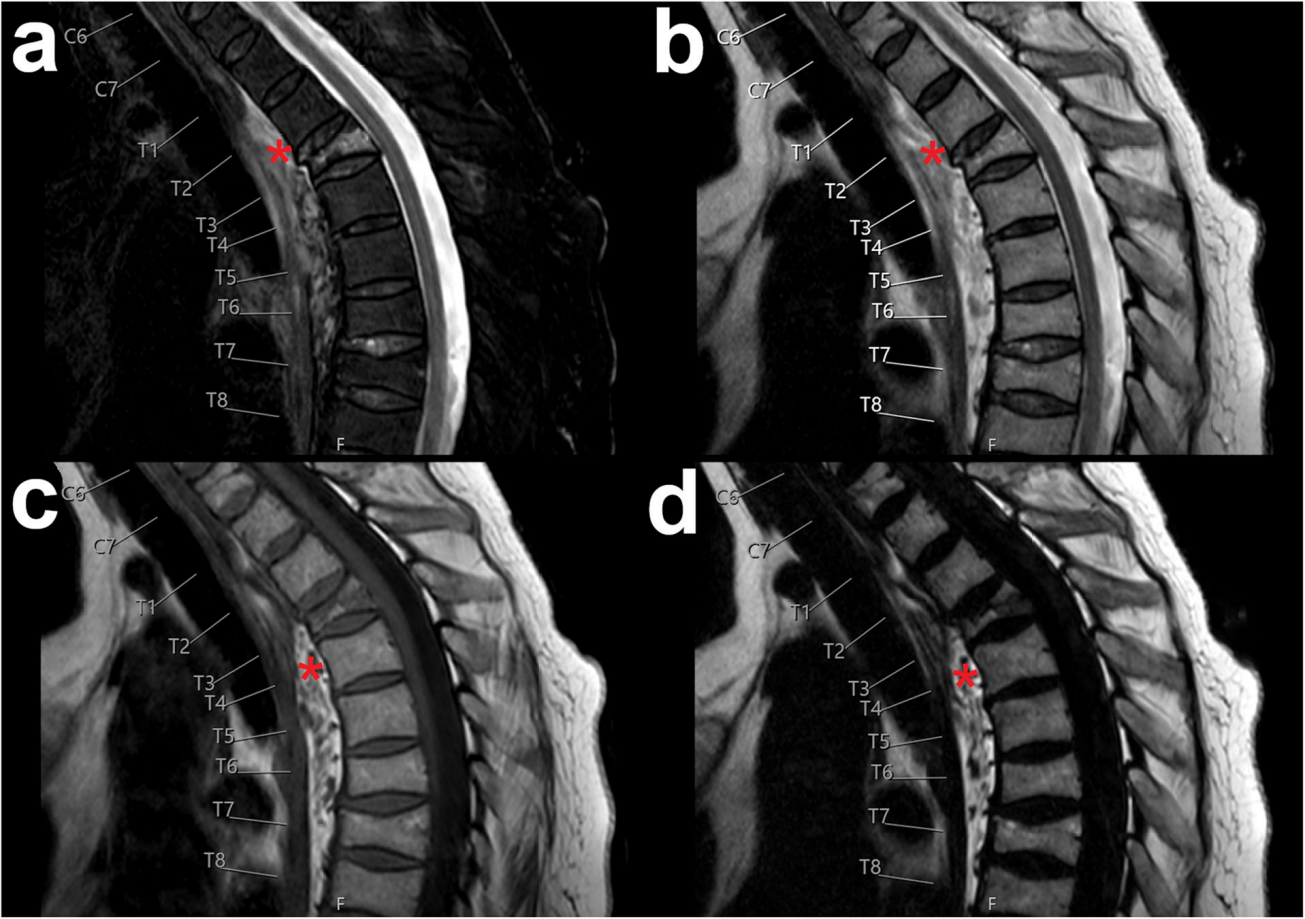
Traumatic thoracic prevertebral haematoma. This figure shows the thoracic spine of a 62-year-old female patient using water-only images (a), in-phase images (b), and fat-only images (d) of the sagittal T2-weighted DIXON sequence, together with a sagittal T1-weighted sequence (c). The patient had suffered a traffic accident and showed a thoracic prevertebral haematoma spanning from T1 to T8 (red asterisks) combined with an acute vertebral fracture (T3)
